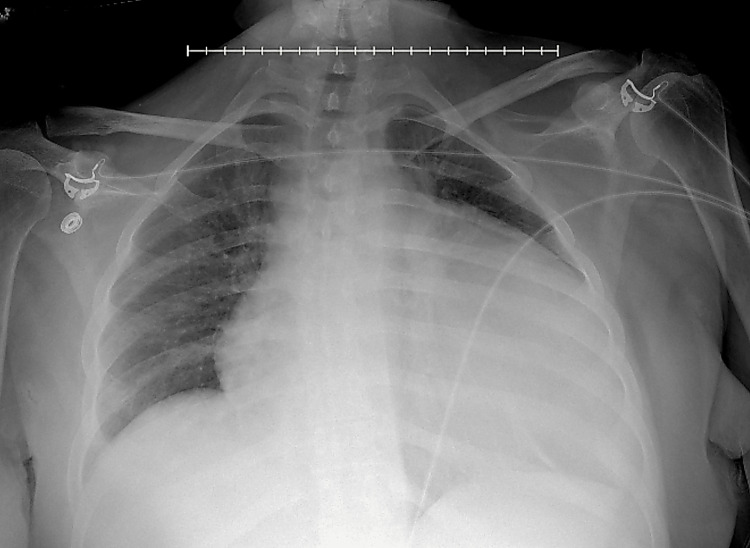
Portable AP chest radiograph demonstrating an enlarged cardiac silhouette. AP: anteroposterior
